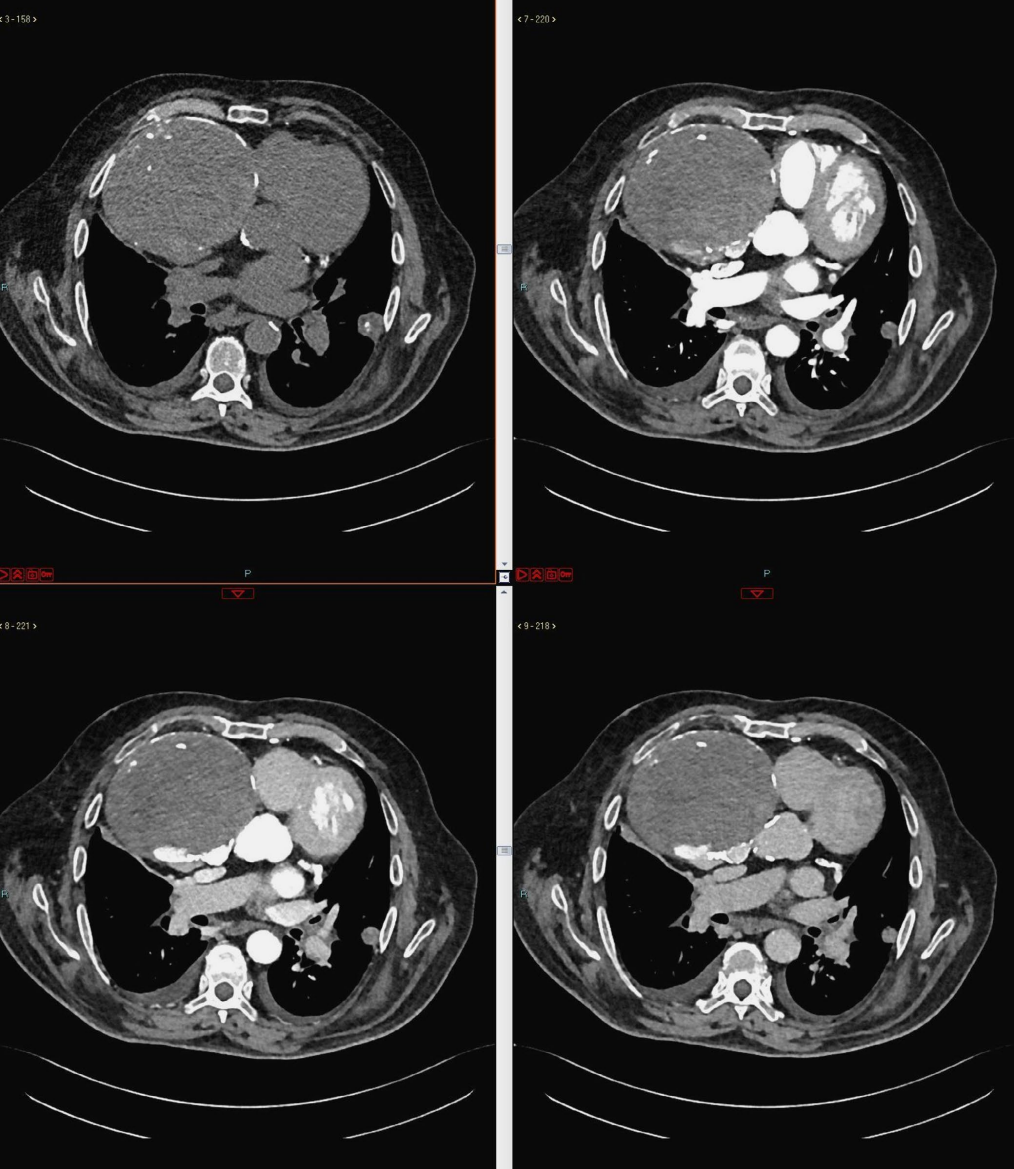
Computed tomography angiography (CTA), axial planes, non‐contrast (upper left), arterial (upper right), venous (lower left), and delayed (lower right) contrast‐enhanced phases. CTA scan shows a large formation (16 × 14 × 13 cm) with parietal and intralesional calcifications, predominantly hypodense in the non‐contrast scan and contrast‐enhanced in the delayed phases, causing right atrium compression.
